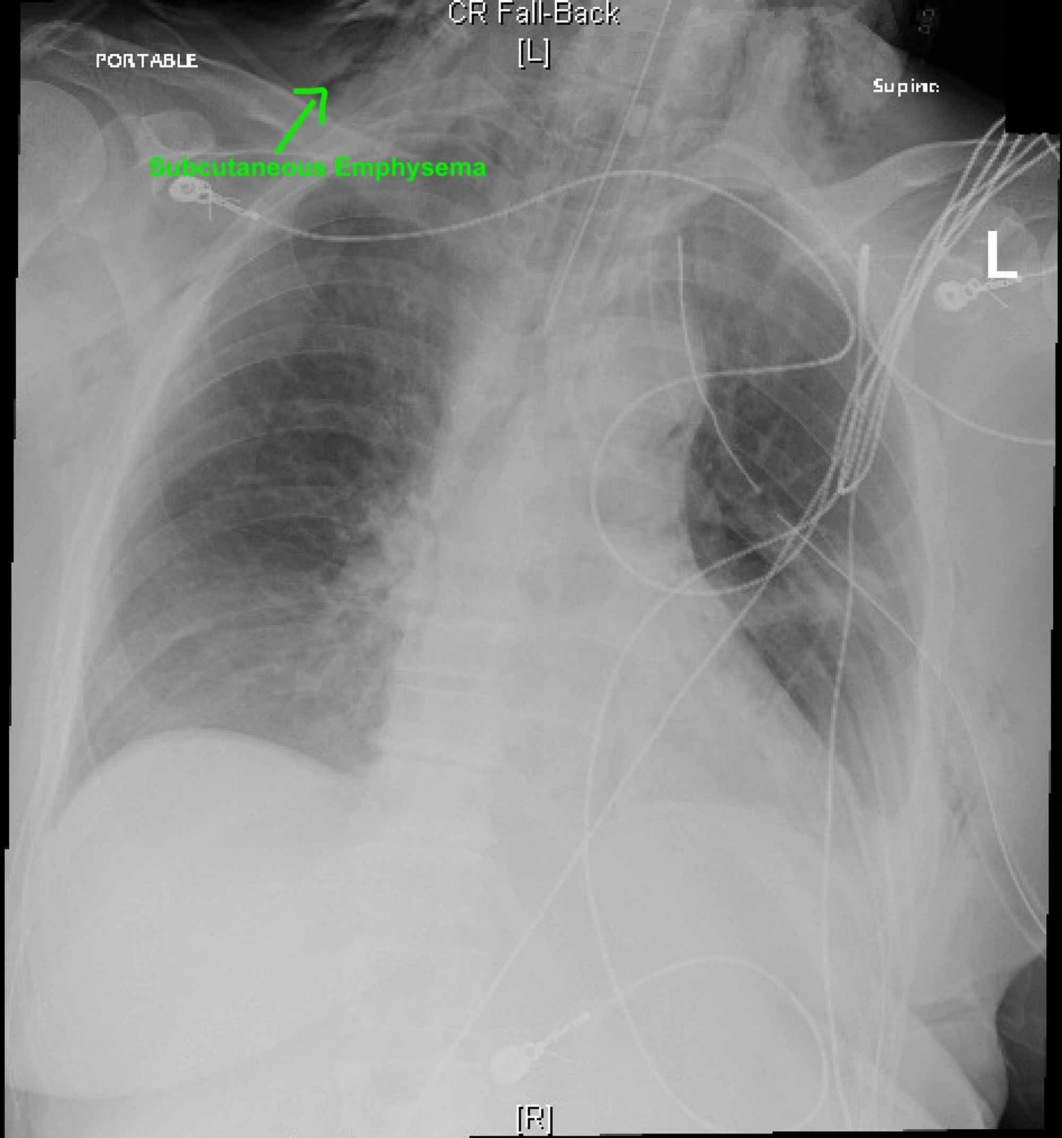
Chest radiograph post endotracheal intubation
